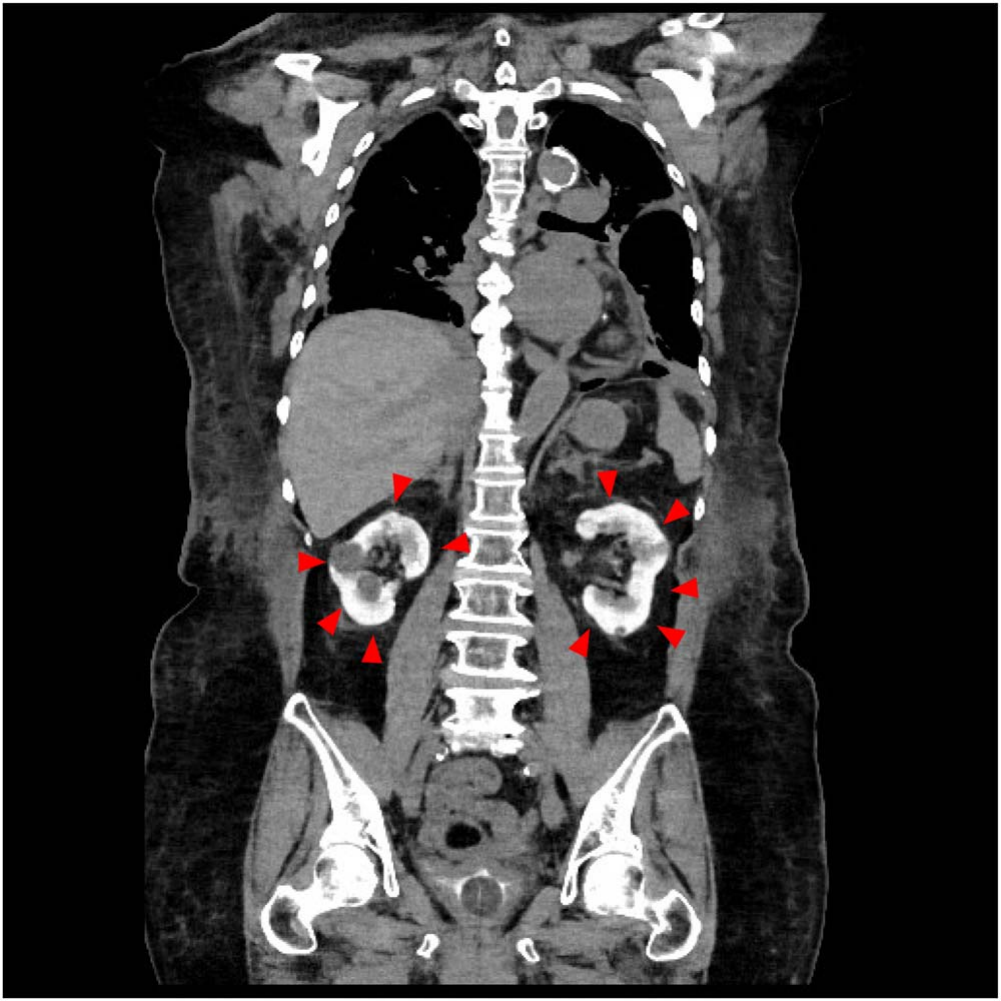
Bright kidney detected by non-contrast computed tomography 4 days after the percutaneous coronary intervention (axial).
